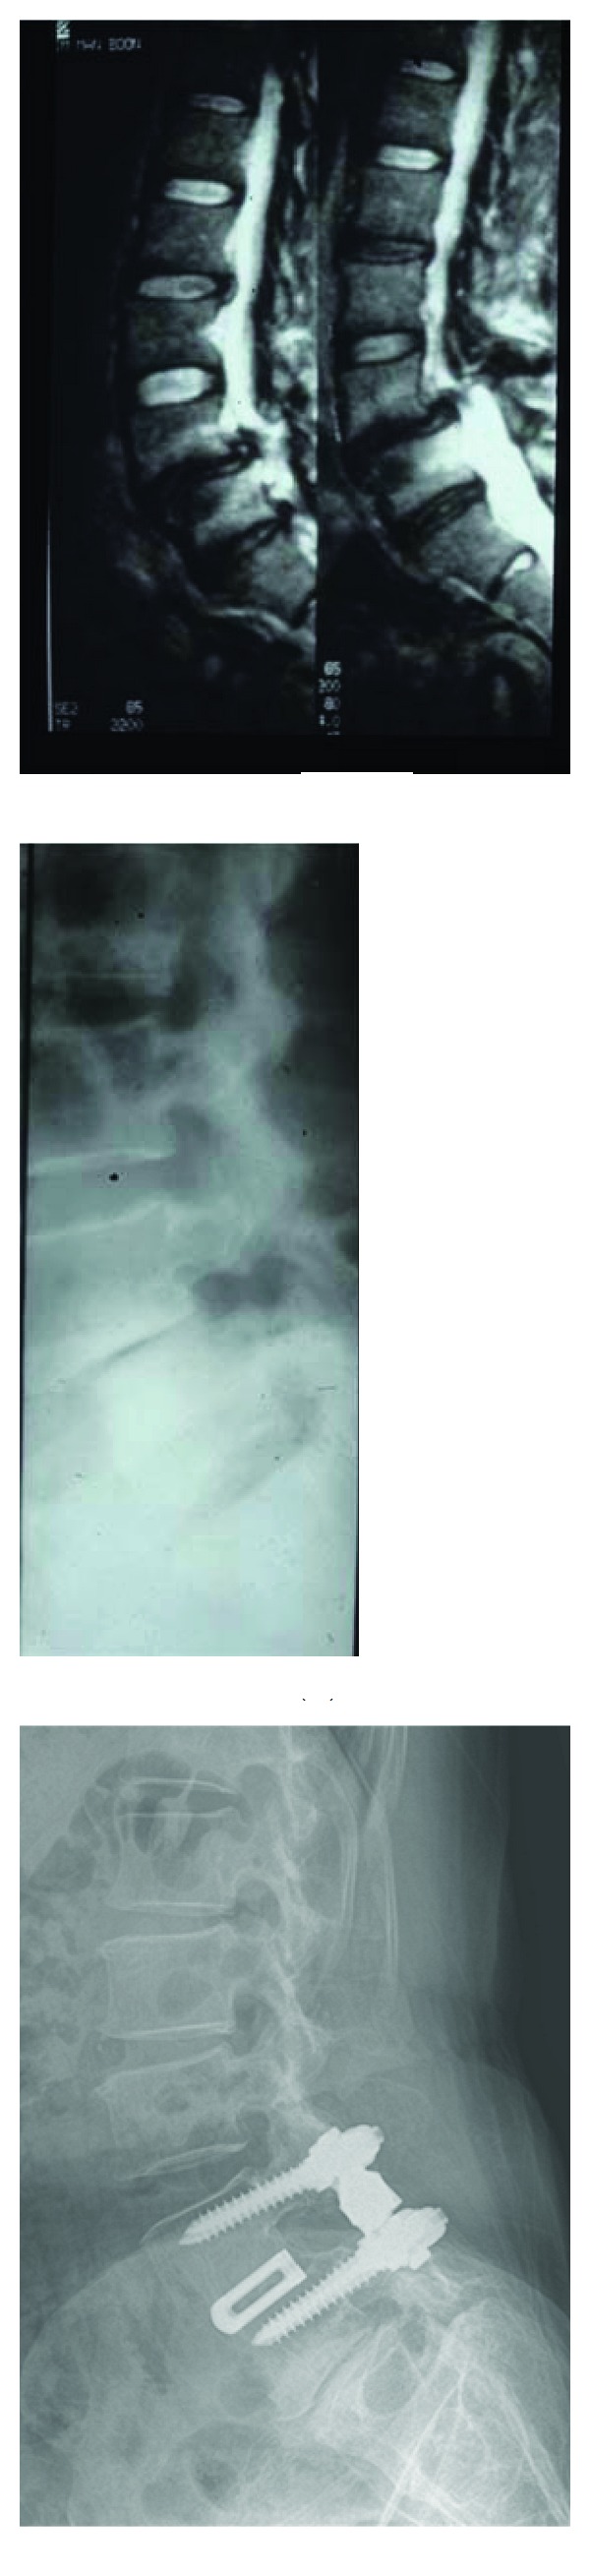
Example of mild degeneration (image at right, compared to preoperative X-ray film and MRI at left) 7 years after surgery in a 67-year-old woman pain-free and satisfied with her result.
